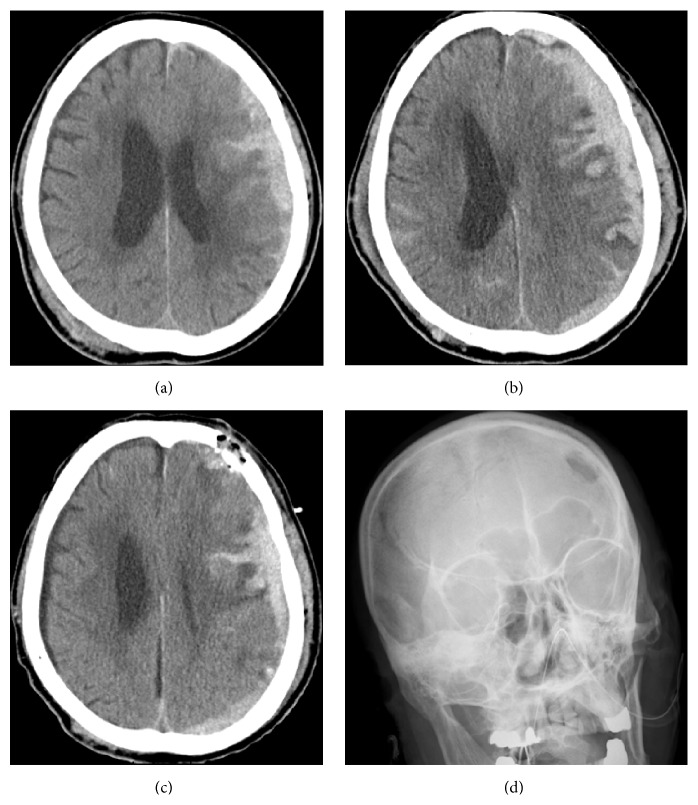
(a) Axial plain CT scan at the time of admission shows left acute subdural hematoma and bilateral frontal contusion with thickness of 14 mm and midline shift of 8 mm. There is a bruised area in the right parietal region without bone fracture. (b) Axial plain CT scan 72 hours after admission shows worsened acute subdural hematoma with thickness of 16 mm and midline shift of 9 mm. Massive contusion of the left frontal lobe has occurred. (c) Radiographic frontal view shows the location of the burr hole 4 cm above the left eyebrow. (d) Axial plain CT scan after surgery shows reduced hematoma. Midline shift had improved to 4 mm. There is a small amount of air in the subdural space. Burr hole is covered by bone powders.
